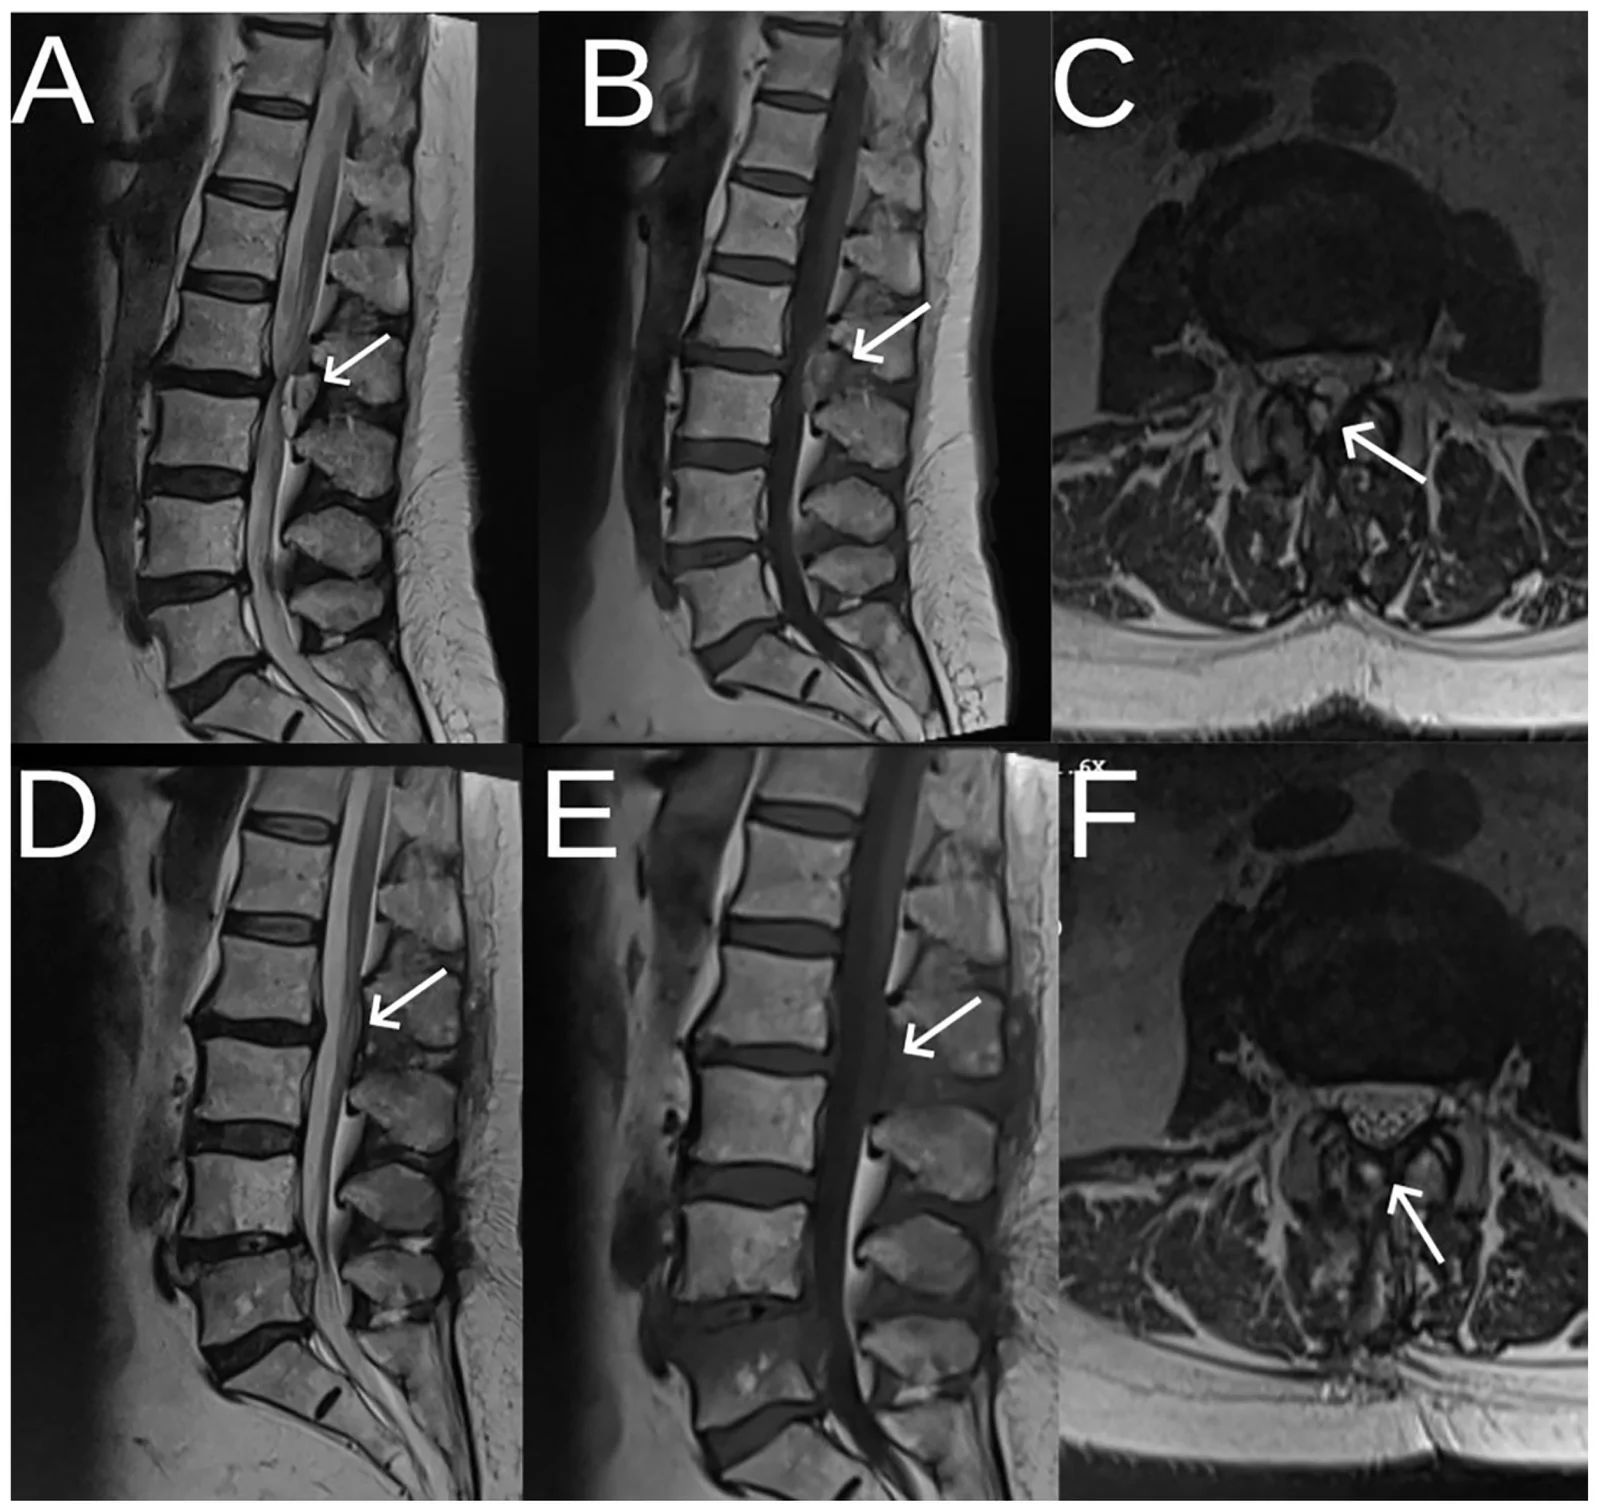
Representative case of chronic spontaneous lumbar epidural hematoma at the L2–L3 level. (A) Preoperative sagittal T2-weighted MRI demonstrating a dorsal epidural lesion at the L2–L3 level causing marked compression of the thecal sac (arrow). (B) Preoperative sagittal T1-weighted MRI demonstrating the dorsal epidural lesion (arrow). (C) Preoperative axial T2-weighted MRI demonstrating significant spinal canal compression by the epidural lesion (arrow). (D) Postoperative sagittal T2-weighted MRI demonstrating complete evacuation of the hematoma and satisfactory decompression of the thecal sac (arrow). (E) Postoperative sagittal T1-weighted MRI demonstrating no residual epidural lesion following surgical evacuation (arrow). (F) Postoperative axial T2-weighted MRI demonstrating adequate spinal canal decompression following hematoma evacuation (arrow).
